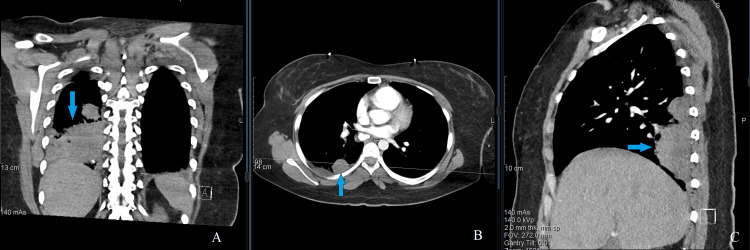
CT chest on admission, in three planes Coronal plane (A), axial plane (B), and sagittal plane (C). Arrows showing lung mass of the right lower lobe measuring 5 × 5 × 3 cm.
